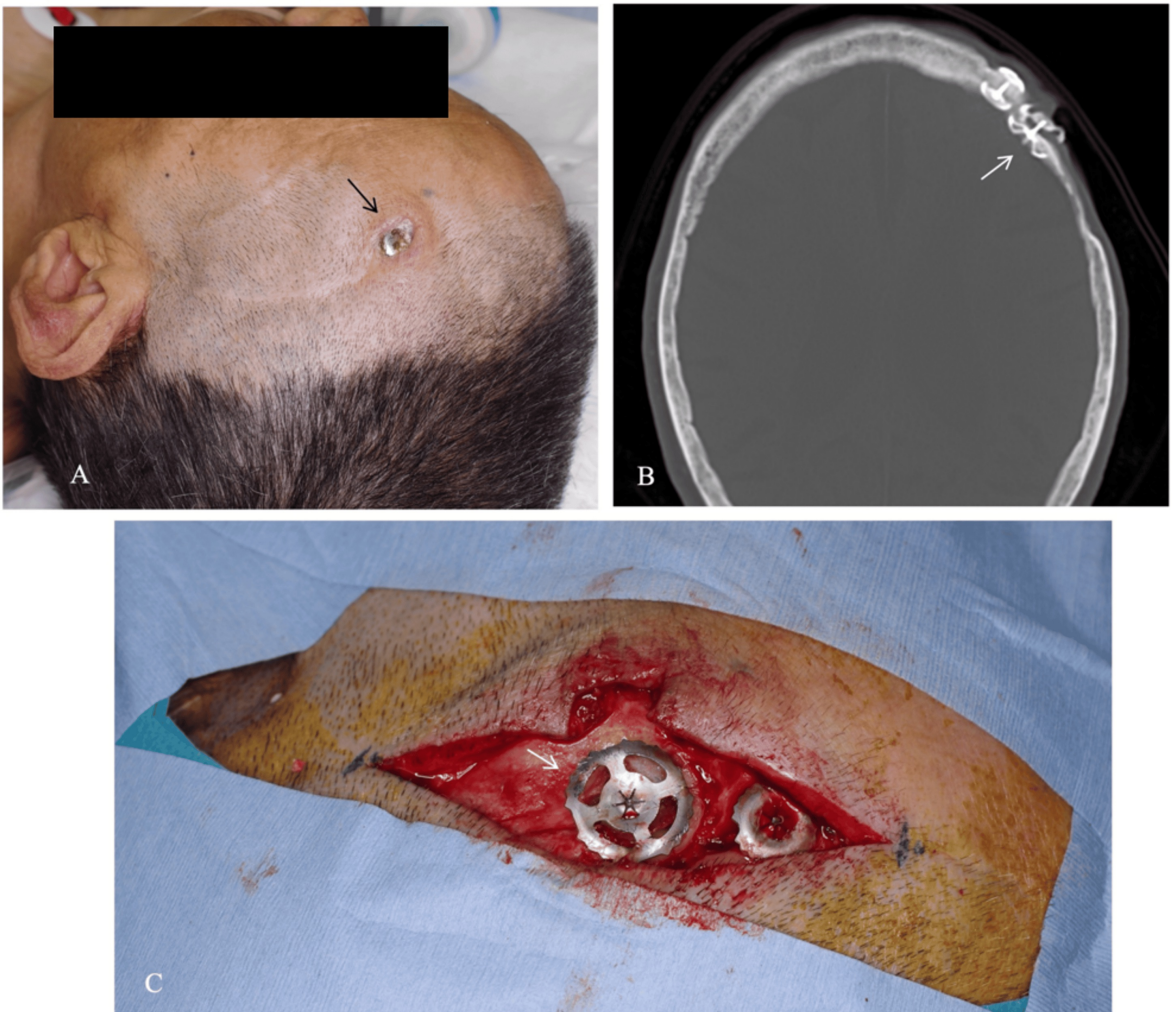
(A) A metallic plate (arrow) is exposed in the left frontal region. (B) CT of the head shows that the exposed hardware at the same site represents a clamp-type metallic plate (arrow). (C) After scalp incision and bone exposure, a metallic plate (arrow), identified as a CranioFix® (B. Braun Aesculap Co., Tokyo, Japan), is confirmed.
